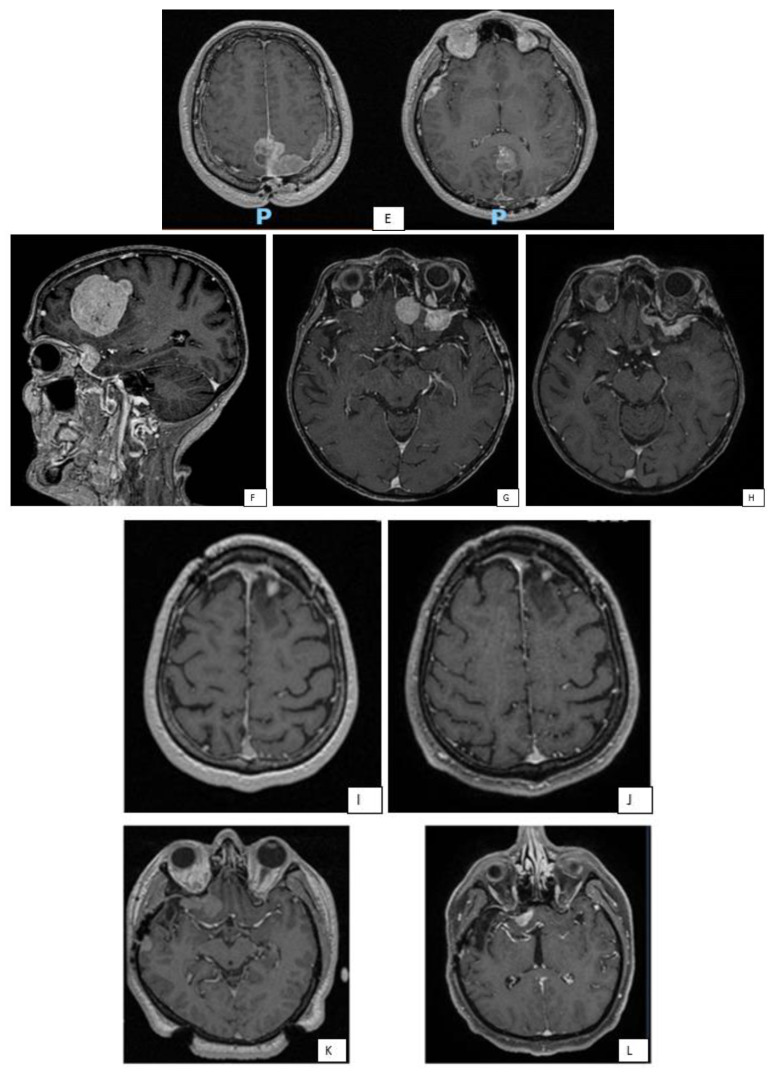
MRI images of some representative cases. Patient with right fronto-parietal convexity meningioma (A), subjected to GTR (Simpson I), as can be seen from the post-operative brain MRI (B). Due to the pathological features (high number of mitoses and cerebral invasion) the patient underwent adjuvant RT treatment despite surgical removal Simpson I. Good local control after two years from adjuvant RT (C). After 36 months, appearance of distal recurrence (D). MRI of a patient with multiple meningiomas (E). Patient with large left frontal meningioma, subjected to surgical removal (F), associated with two other nodules at the left sphenoidal wing, subjected to GKRS (G). Picture (H) shows good disease control 4 years after GKRS treatment. GKRS for a small recurrence of left frontal convexity meningioma (I) and relative MRI control 18 months after (J). GKRS for two nodules of right fronto-temporal meningioma (K). 15 months after radiosurgery, a new GKRS was performed on a further nodule of recurrence at the sphenoidal wing (L).
